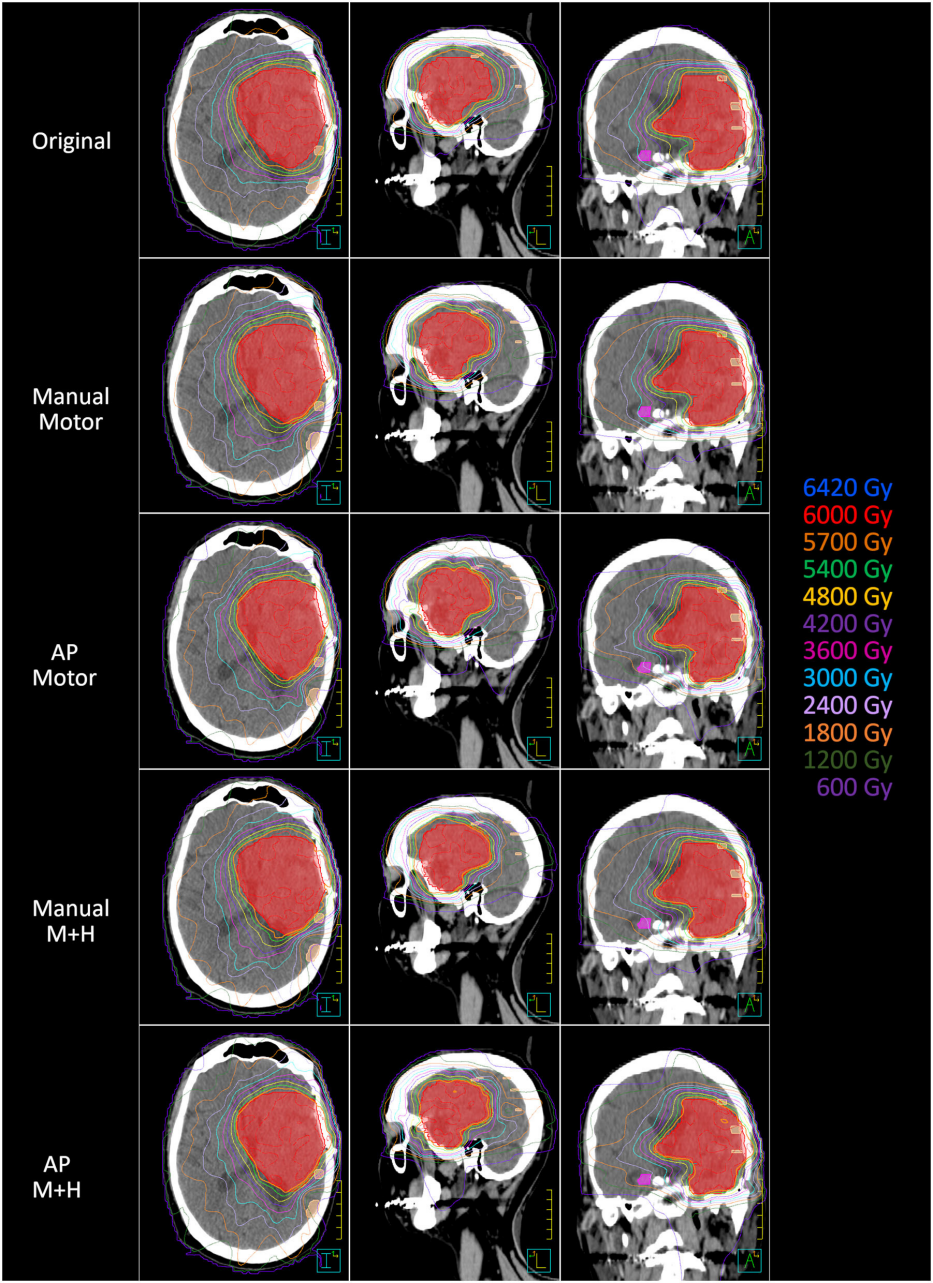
Example of the dose distribution in the planning CT (axial, sagittal, and coronal axis) (left, middle, and right). PTV = red color, motor cortex = skin color, contralateral hippocampus = pink color.
